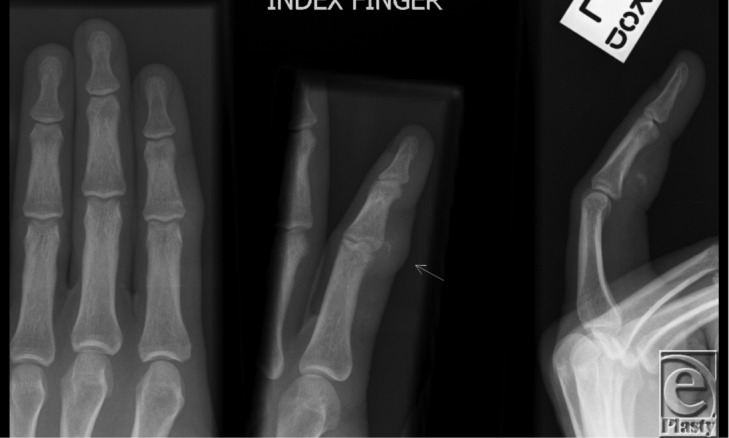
Initial radiograph showing soft-tissue prominence with a mostly radiolucent lesion with peripheral calcification volar to the base of the middle phalanx.
